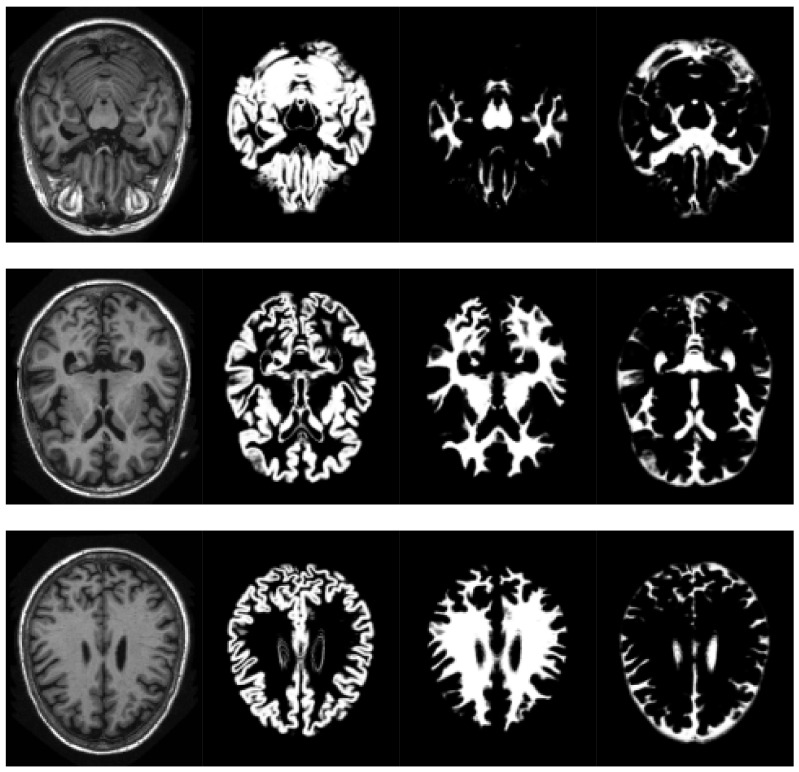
Volume slices, selected from the pre-processed OASIS-1 dataset, showing tissue segmentation examples. Original T1-weighted MRI slice (left), gray matter probabilities (middle left),white matter probabilities (middle right), cerebrospinal fluid (right).
